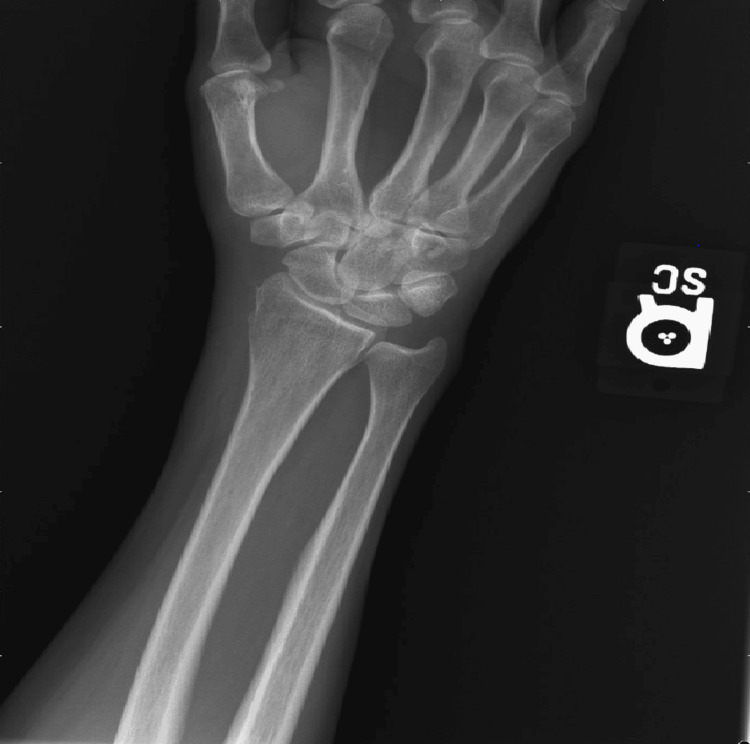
Right wrist X-ray Alignment is anatomic without fracture or lytic lesion. Joint spaces are well preserved. Soft tissues are normal. There is no cortical destruction or periosteal reaction to suggest plain film evidence of osteomyelitis.
